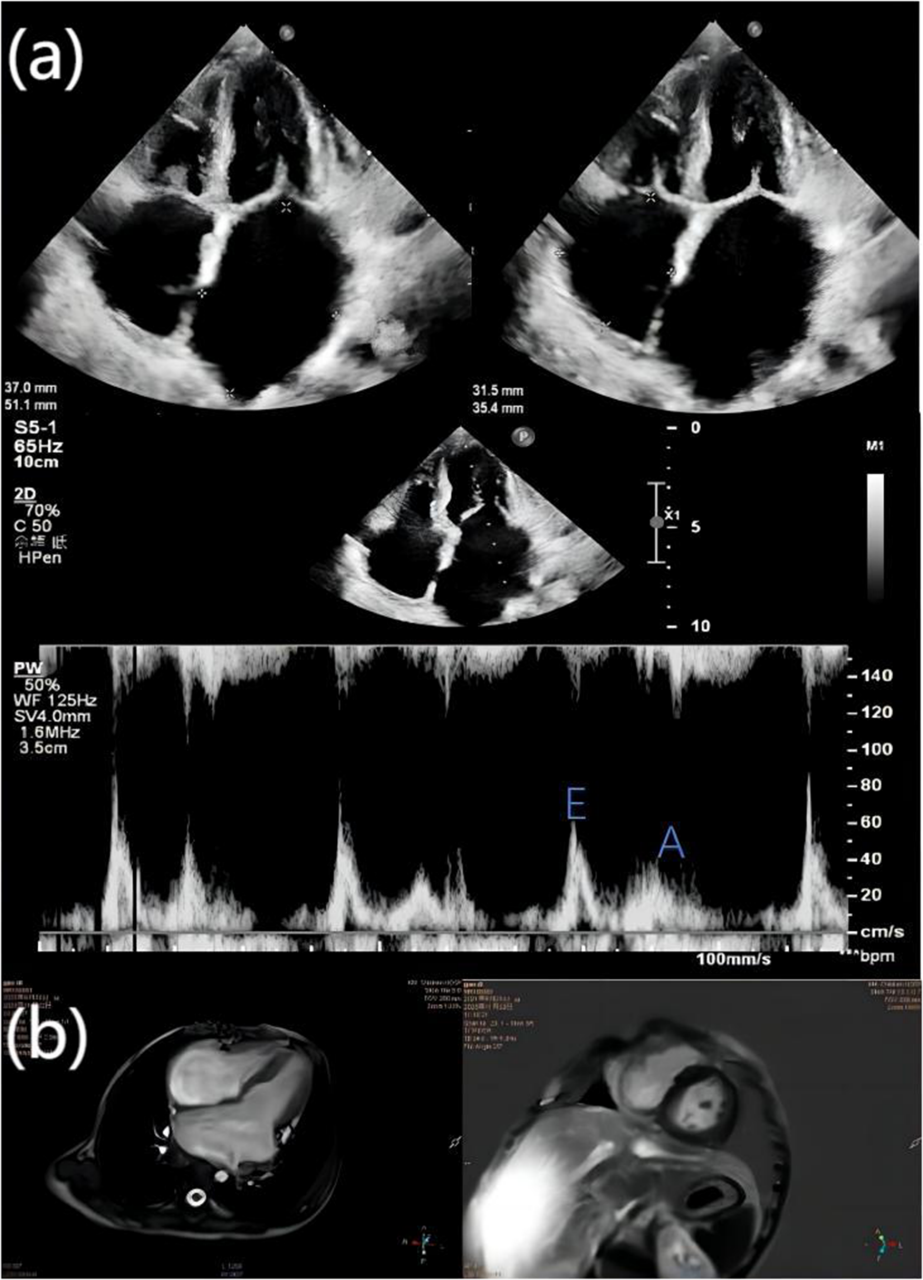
(a) echocardiography showed bi-atrial enlargement, left atrium diameter 37 mm × 51.1 mm, right atrium diameter 31.5 mm × 35.4 mm, right and left ventricular diastolic hypoplasia, normal septal and ventricular wall thickness, and trace pericardial effusion; the ratio of the peak E and peak A velocities of mitral inflow (E/A) was 2.05. The mitral inflow was 2.05%, and the peak A velocity was 2.05%. (b) The cardiac magnetic resonance four-chamber view of the heart shows bi-atrial enlargement and ventricular narrowing, and gadolinium-delayed enhancement in both chambers of the heart shows suspicious striations.
